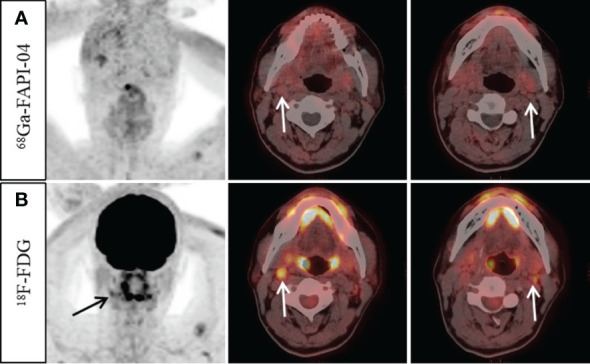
A 57-year-old man with nonkeratinizing undifferentiated carcinoma. An increase ^18^F-FDG uptake was observed in the bilateral cervical (level II) lymph nodes (B, arrow, SUVmax 3.3–4.2). However, no abnormal ^68^Ga-FAPI-04 uptake was observed in the cervical lymph nodes (A, arrow). Ultrasound-guided biopsy of the right level II lymph node revealed proliferating lymphoid cells with no signs of metastatic disease. Finally, it was confirmed by follow-up that all the suspected metastatic lymph nodes were reactive.
